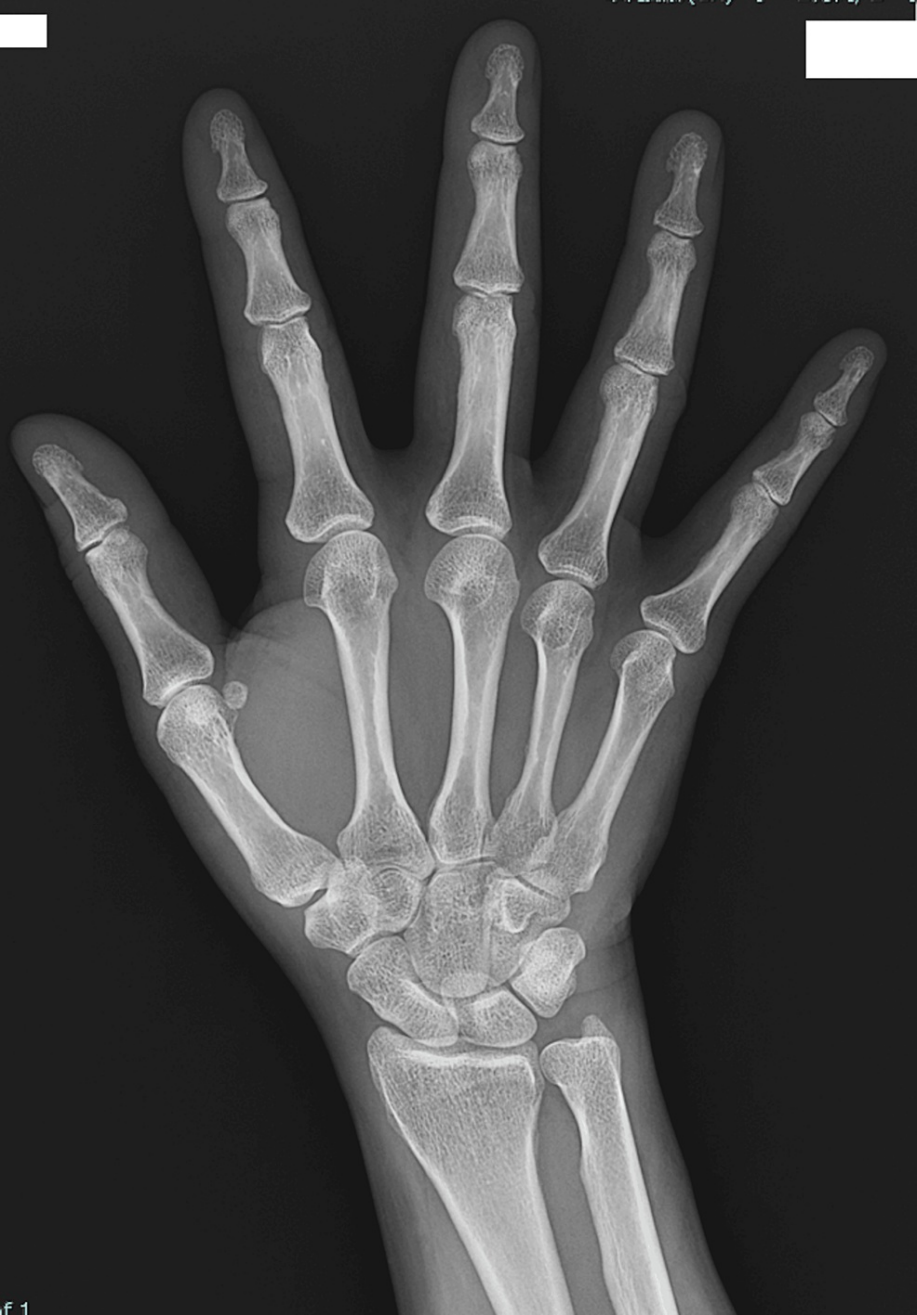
X-ray of the right hand No findings suggestive of a rheumatoid joint were noted.
